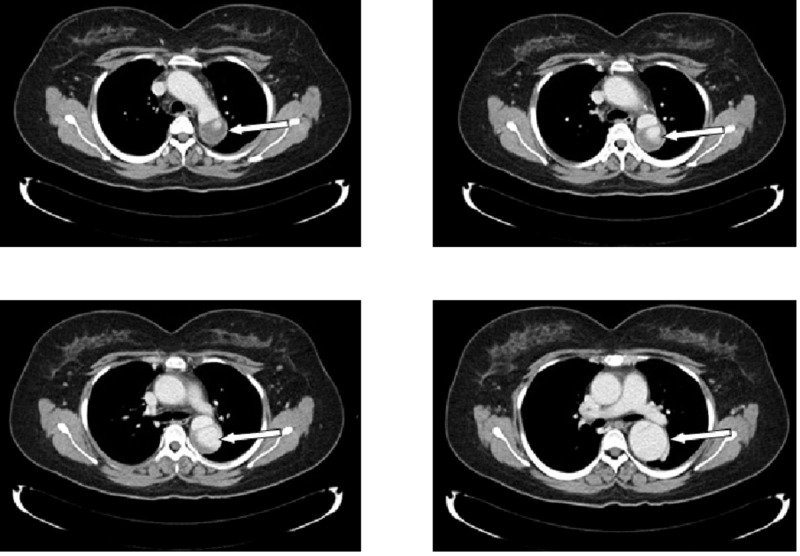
Computed tomography (CT) scan showing a dissecting aortic aneurysm (white arrow), 2014.
